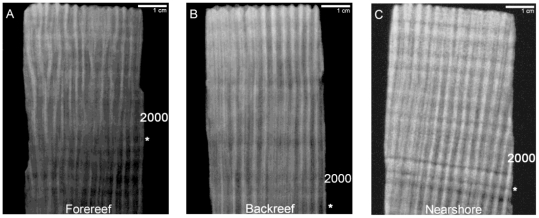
X-radiographs of sample coral cores. Core sections represent the most recent years of skeletal extension for *Siderastrea siderea* from the (A) forereef [core FR-12], (B) backreef [core BR-06], and (C) nearshore reef [core NS-14]. Numbers correspond to year of paired high-low density annual growth bands. Asterisks correspond to the annual growth bands formed during the 1998 coral bleaching event.
